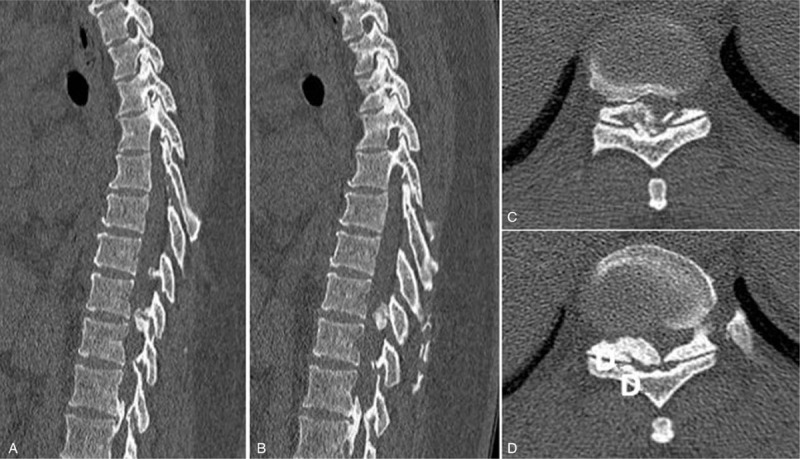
Preoperative CT: T9 and 10 ossification of the ligamentum flavum with dural ossification.
